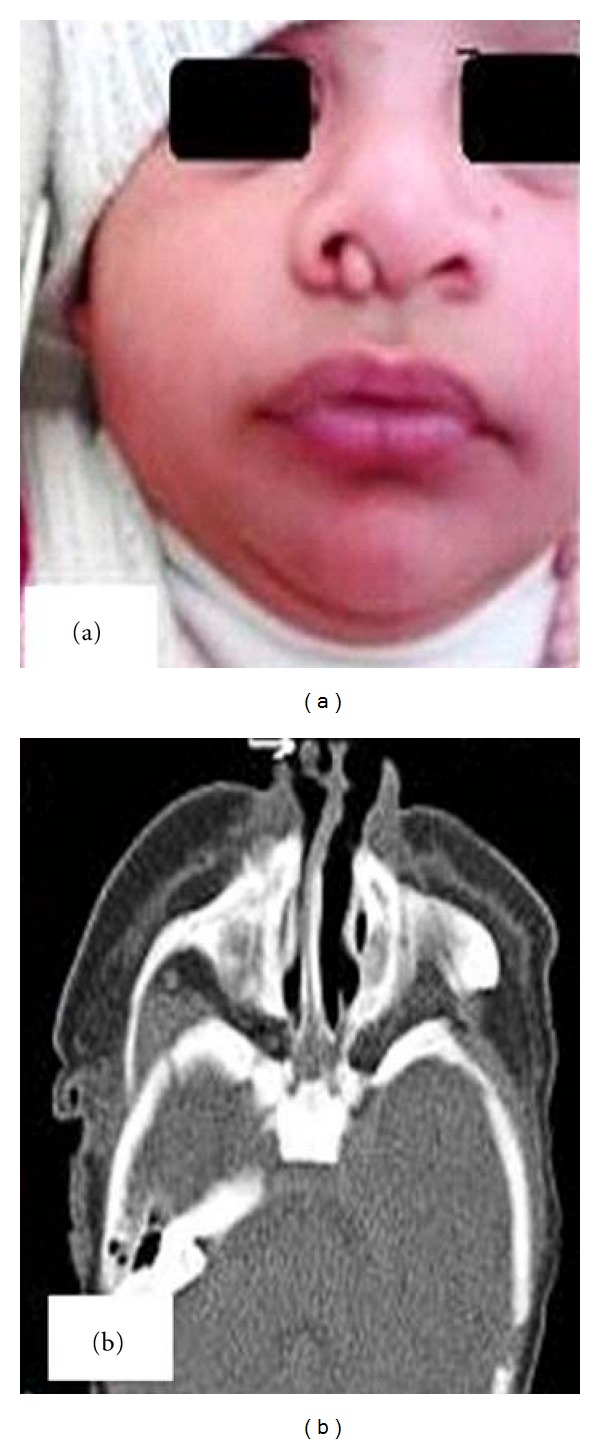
(a) 3 months old baby with right nasal congenital polyp-glioma. (b) Axial CT scan revealing a soft tissue lesion arising from nasal septum (white arrow).
